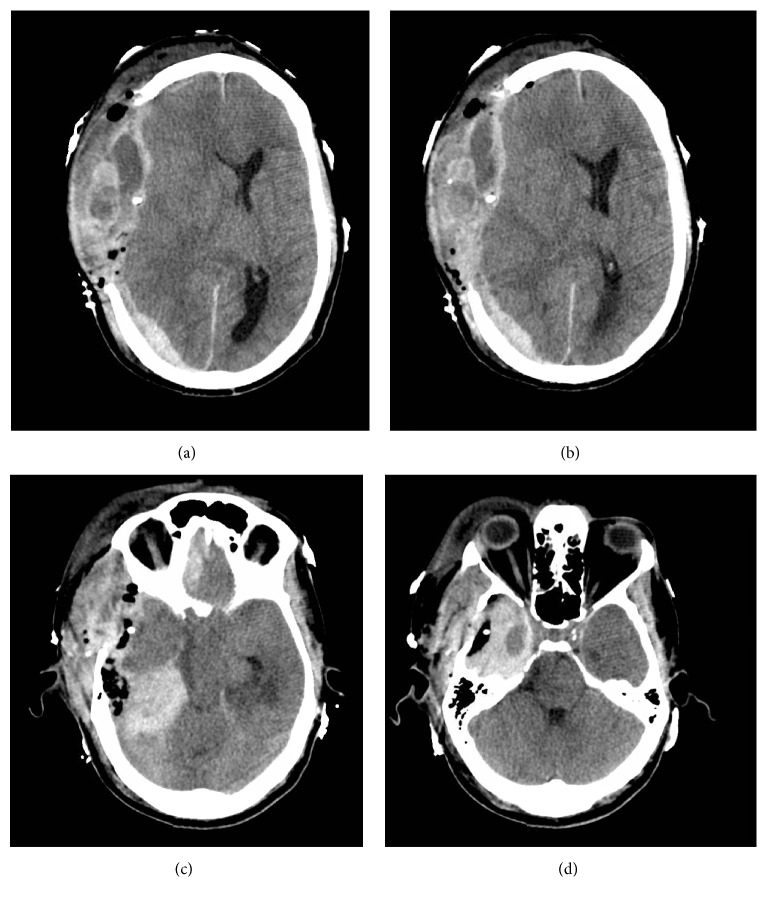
Repeat CT 6 hours after first surgery showing reappearance of mixed density mass along the right convexity with again persistent midline shift ((a) and (b)). There is reherniation with loss of right temporal horn and basilar cisterns once again (c). Of note, the imaging characteristics of the small subtemporal hematoma are unchanged (d), speaking to the differing contents of the panhemispheric subdural density (versus hematoma) seen in panels (a) and (b).
